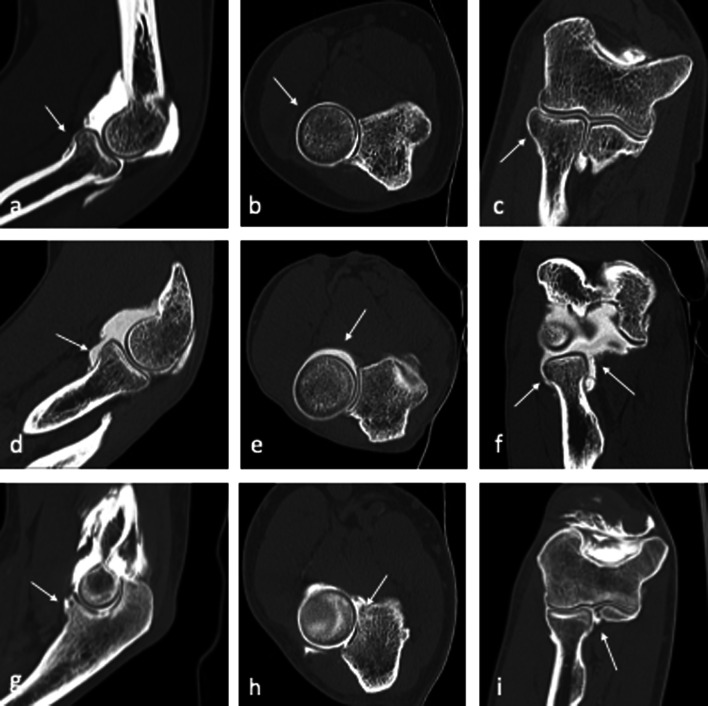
CT-arthrography sagittal (a, d, g), axial (b, e, h) and coronal (c, f, i) images belonging to three patients, two of which display different SMILE stages involving the AL (white arrows). Intact annular ligament (AL) of the lateral collateral ligament (LCL) complex (a–c). Loose AL with no evidence of fiber tearing, displaying the “loose collar sign”, suggestive of patholaxity (d–f). Thickened and irregular AL showing a partial-thickness tear (g–i); ligament fibers appear delaminated as iodinated contrast permeates through their layers
